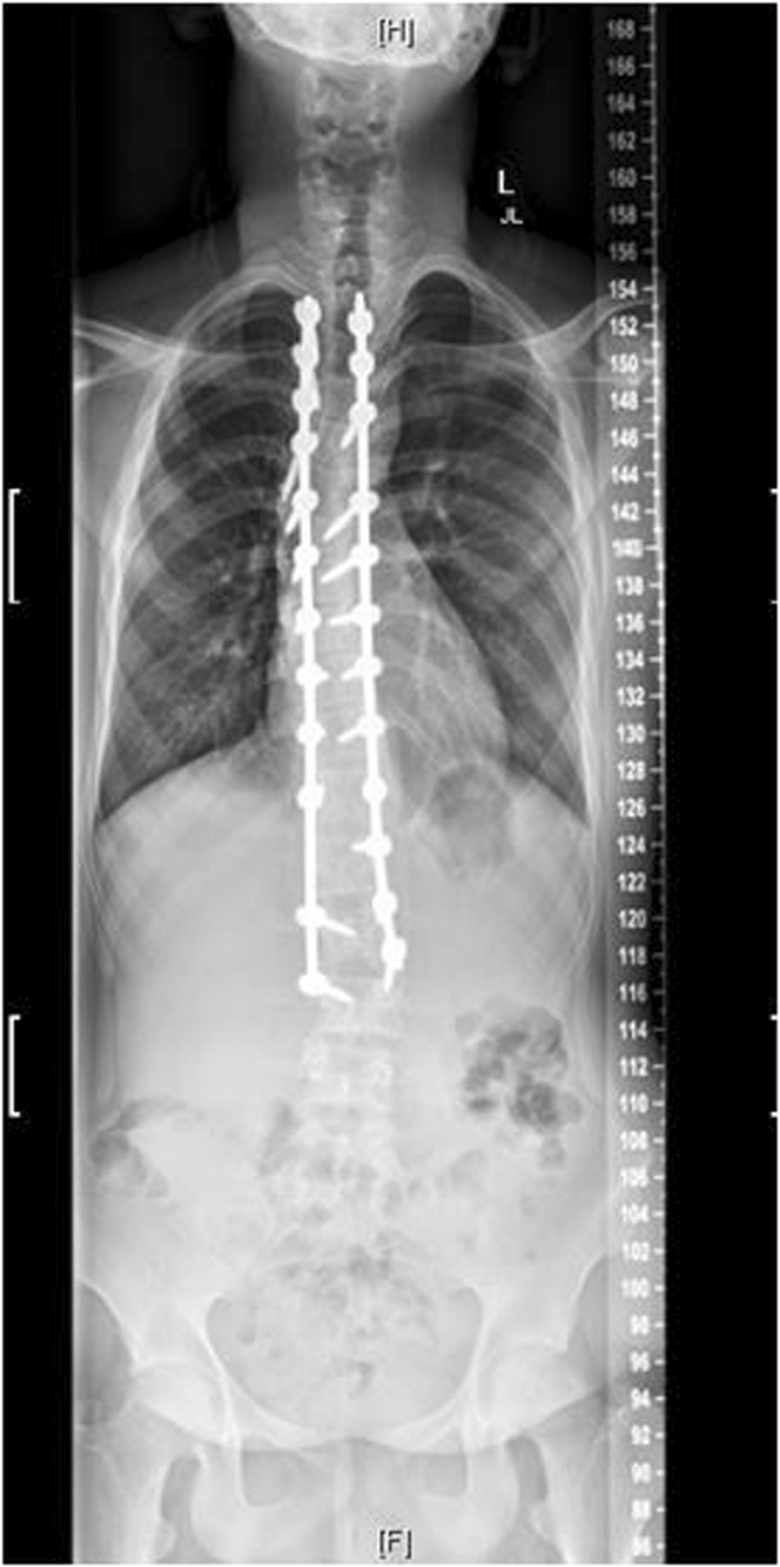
Post-operative radiograph of the same 15 year-old male.
